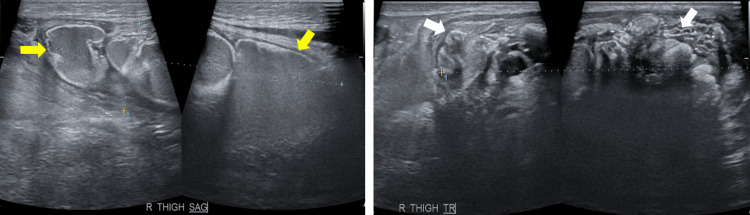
Ultrasound of the right superomedial thigh mass Ultrasound of the right superomedial thigh mass showing cystic (yellow arrows) and solid (white arrows) components of the mass. The mass has a conglomerate measurement of about 9.4x7.7x2.4 cm (CCWAP).
